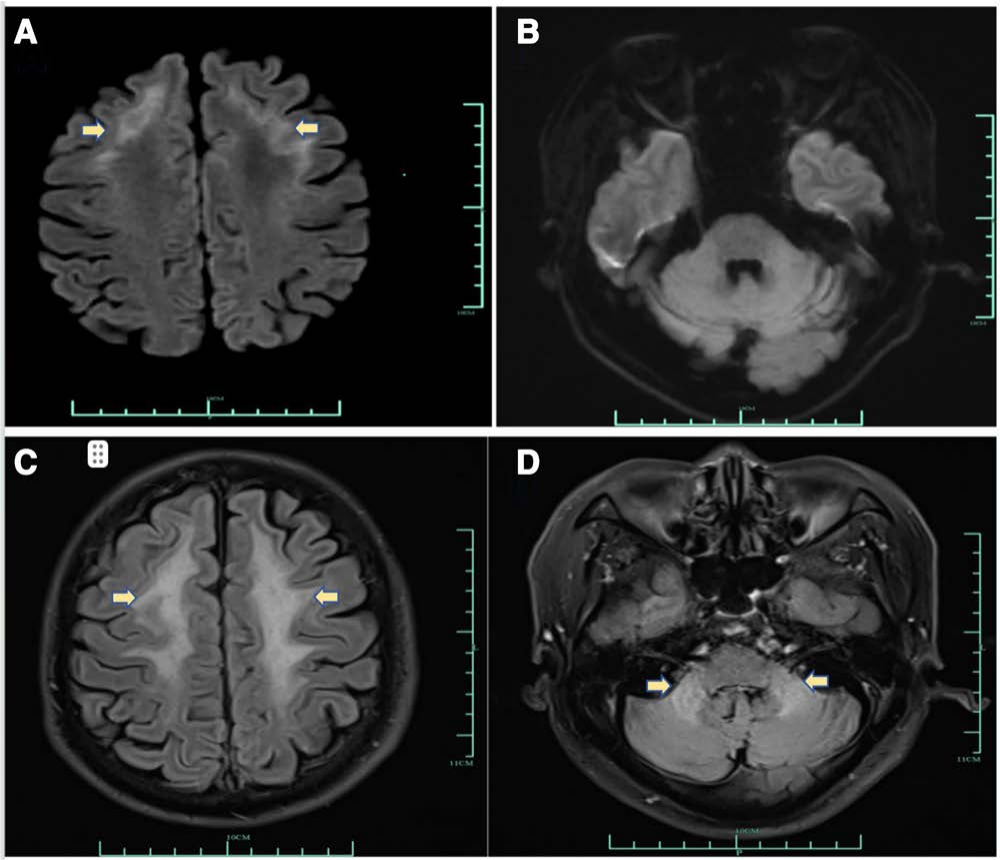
MRI of the brain. (A) MRI diffusion-weighted imaging (DWI) showing hyperintensities in the bilateral frontoparietal corticomedullary junction (yellow arrow).(B) MRI diffusion-weighted imaging (DWI) showing isointensity in the bilateral middle cerebellar peduncles. (C) MRI T2-fluid attenuation inversion recovery (T2-FLAIR) showing hyperintensities in the bilateral frontoparietal cortico-medullary junction (yellow arrow). (D) MRI T2-fluid attenuation inversion recovery (T2-FLAIR) showing hyperintensities in the bilateral middle cerebellar peduncles (yellow arrow).
